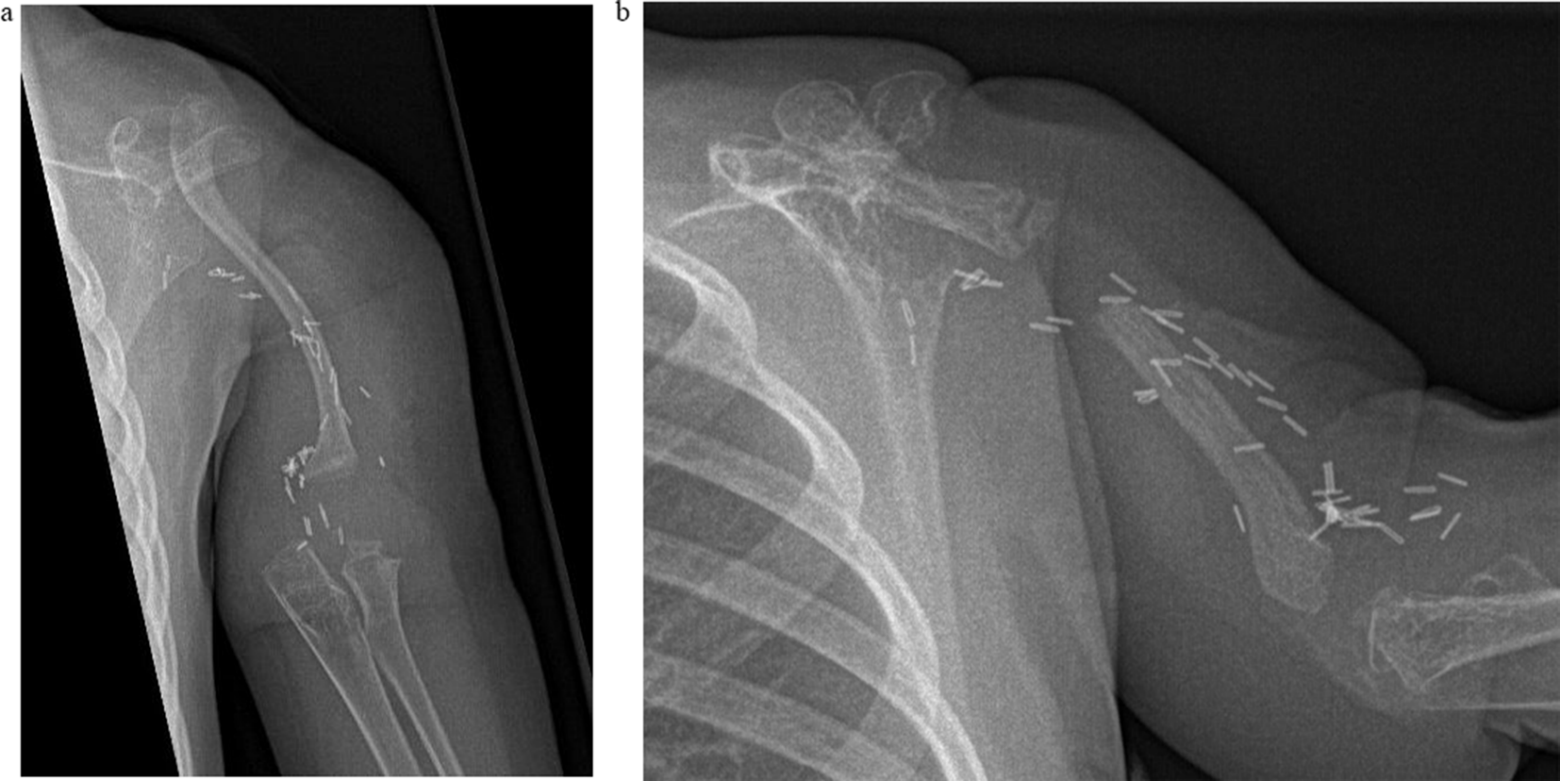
Postoperative radiographs after CPH reconstruction. Fracture and osteolysis were incidentally observed 12 months after the reconstruction (a). Constant presentation of a central osteolysis of the clavicle while retaining arm length and stability in both joints (b) (patient#4)
